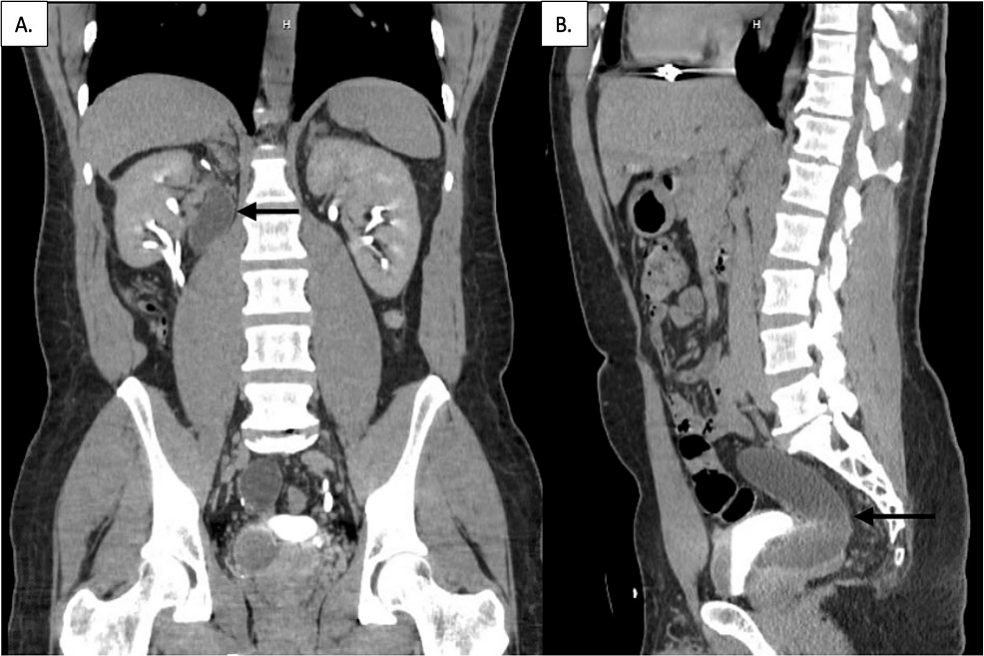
CT abdomen and pelvis with contrast three years post-operatively (A) Coronal view of the right kidney with resolution of hydronephrosis. Notice the dilated ureteral remnant adjacent to the kidney, marked by the black arrow. (B) Sagittal view of right ureter inserting into the posterior prostatic urethra demonstrating worsened hydronephrosis (black arrow).
